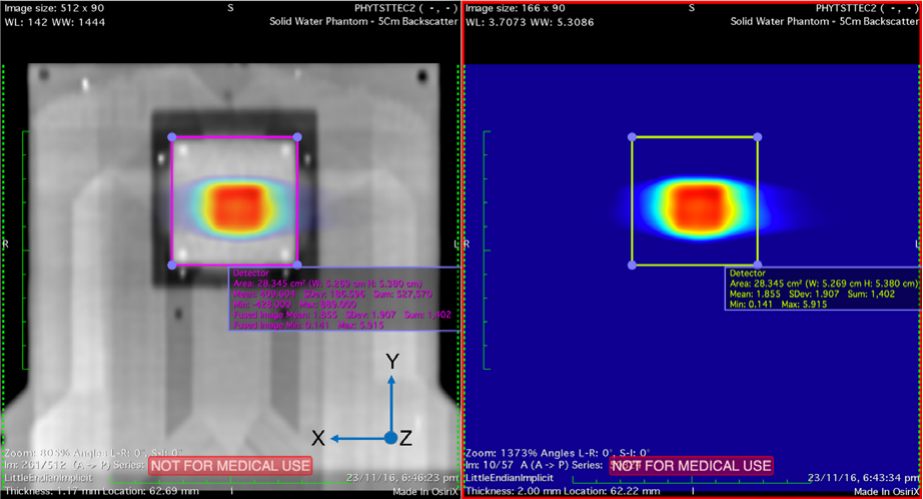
2D dose map extracted from Eclipse TPS overlaid on CT slice of MP512. Boxes highlight the sensitive area of the detector and represent the dose map region used for gamma analysis comparison (coronal plane views).
